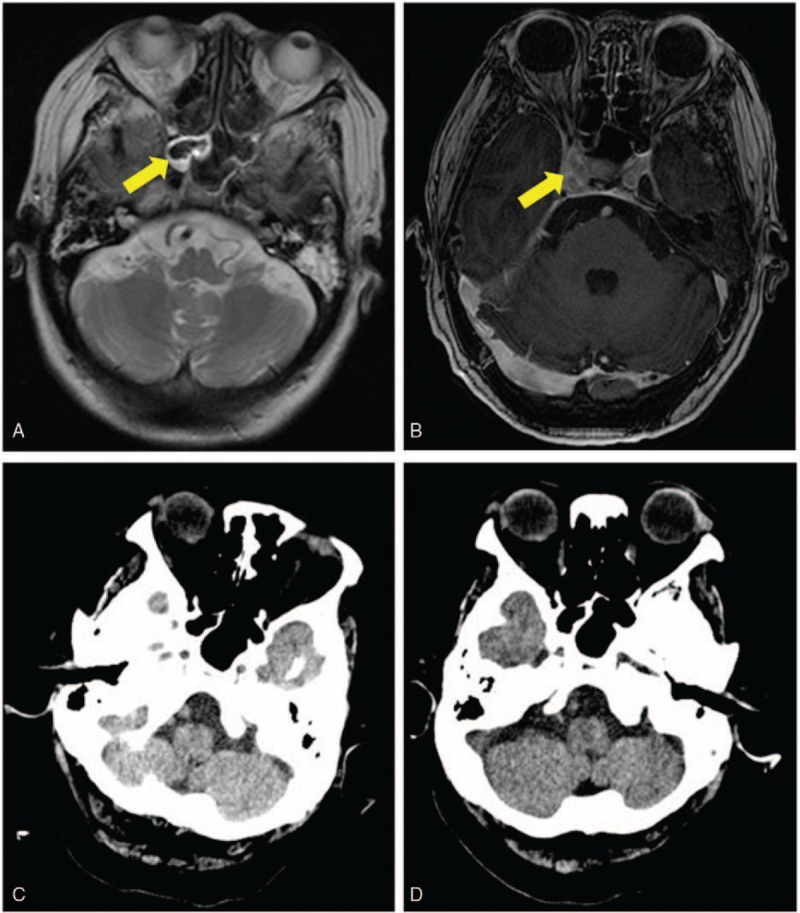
Sphenoid sinus display in MRI and CT: (A) On day 59, the right sphenoid sinus wall shows hyperintense signal on T2-weighted MR images (arrow marked). (B) On day 64, contrast-enhanced MR images shows abnormally enhancing soft tissue in the right sphenoid sinus (arrow marked). CECT on day 63 (C) and CT on day 76 (D) does not reveal the sphenoid sinus disease. CECT = contrast-enhanced computed tomography, CT = computed tomography, MRI = magnetic resonance imaging.
